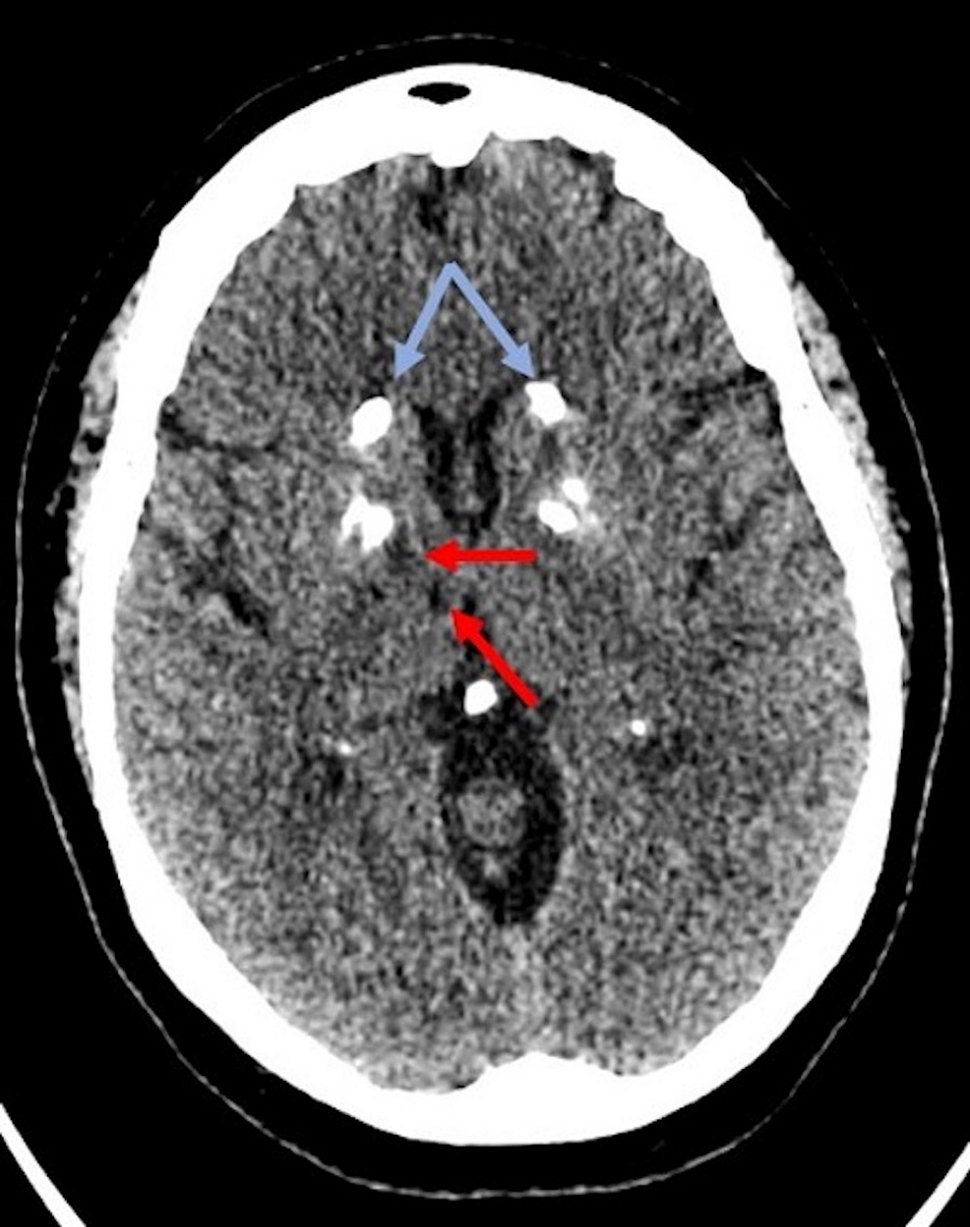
Non-contrast axial CT image of the head demonstrating subtle foci of hypo-attenuation in the region of the anterior right thalamus (red arrows). Bilateral basal ganglia calcifications are noted (blue arrows).
